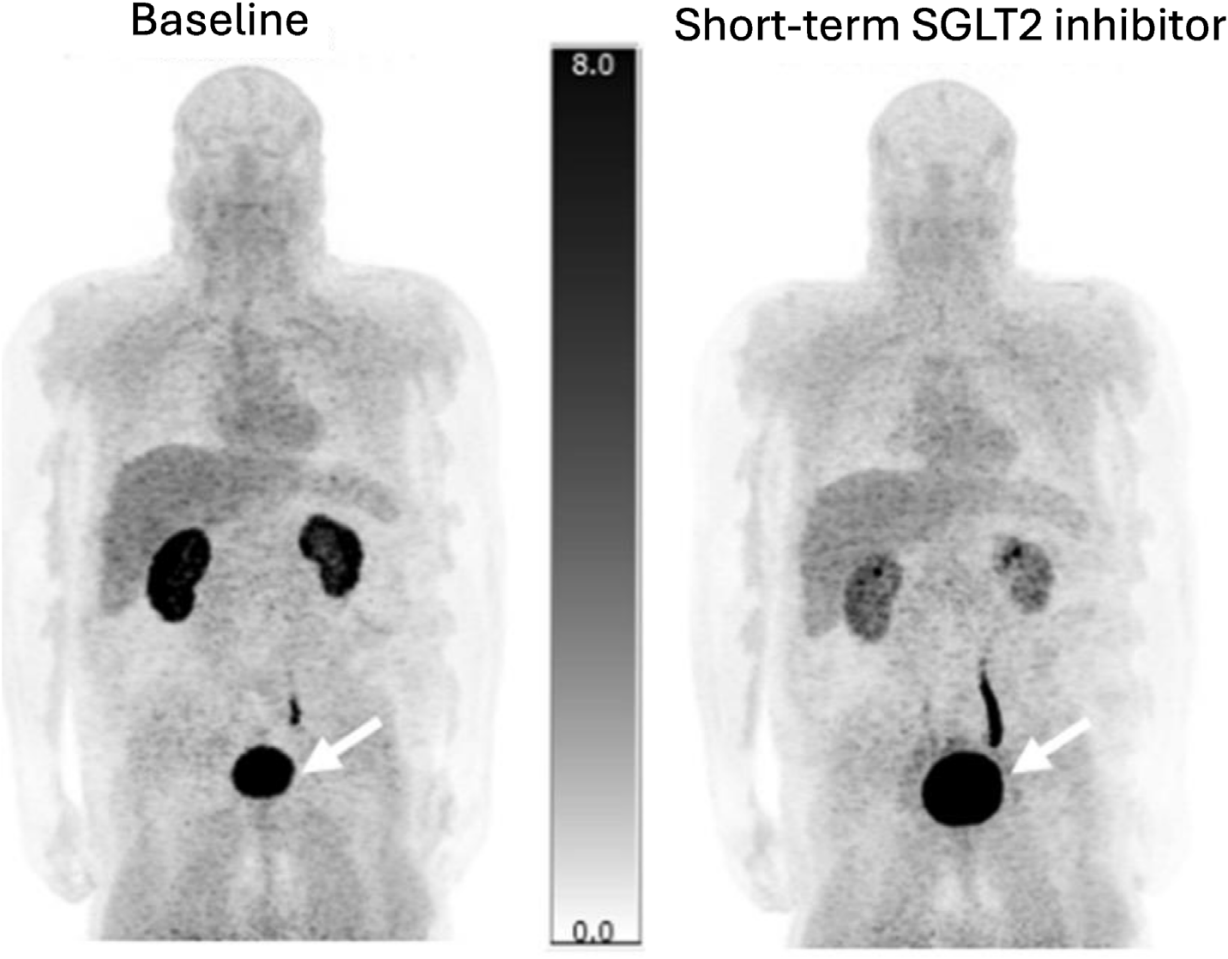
Whole-body PET imaging with ^18^F-Me-4FDG in a type 2 diabetes mellitus patient demonstrates a decrease in renal uptake alongside increased bladder accumulation (indicated by white arrows), comparing baseline (left) and post-2-week treatment with an SGLT2 inhibitor (right). Adapted with permission [48], Copyright 2023, Springer Nature.
